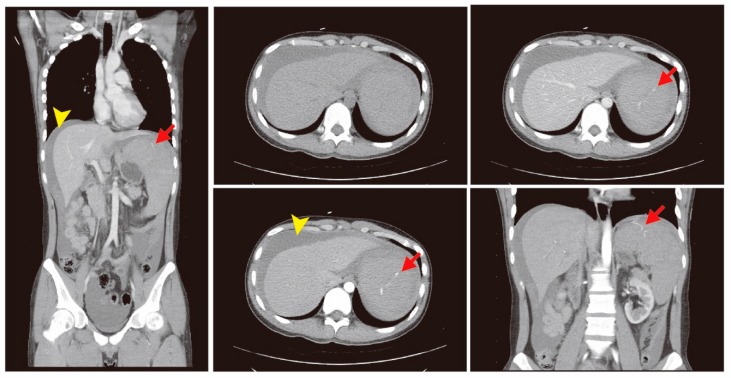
The abdominal contrast enhanced computed tomography (CT) showed splenomegaly and a splenic rupture (red arrow) with hemoperitoneum (yellow arrow head).
